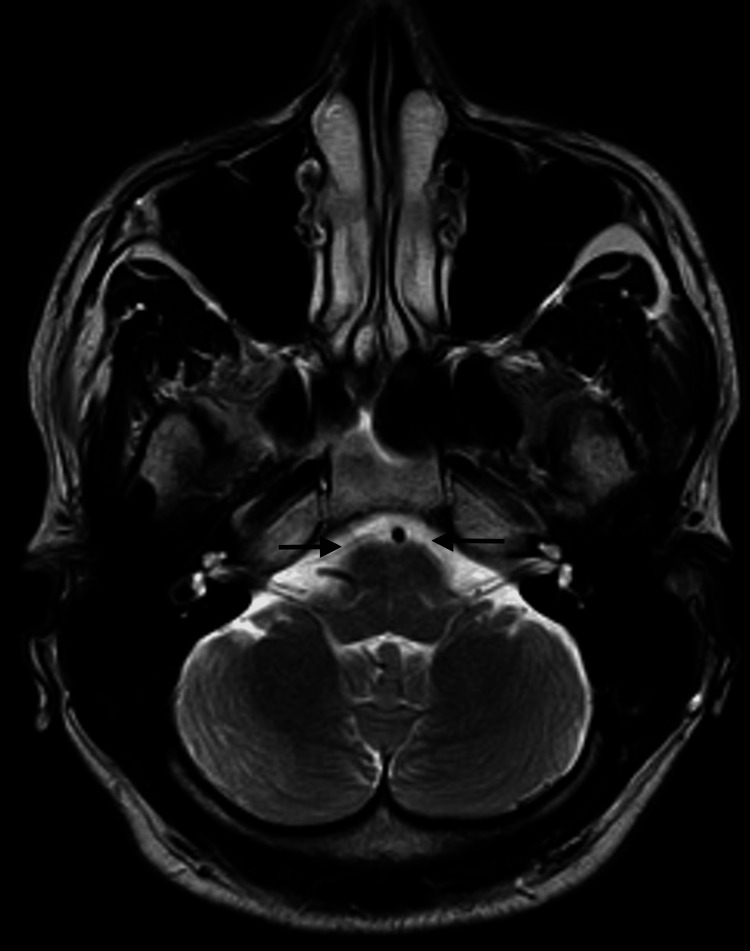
Contrast-enhanced MRI: A follow-up study. Axial T2 bulb follow-up. Slight improvement in bilateral and symmetrical hyperintensity of the mesencephalic tectum and, to a lesser extent, the bulbar tectum is observed on T2-weighted images (arrows).
